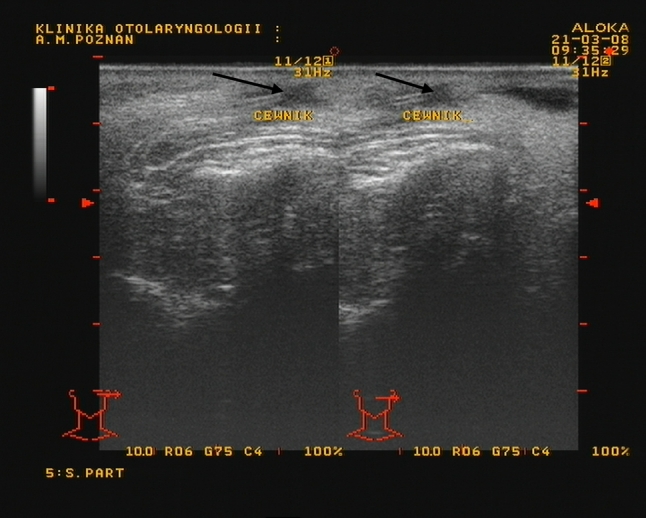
The position of the stent in ultrasound examination (*arrows*)
